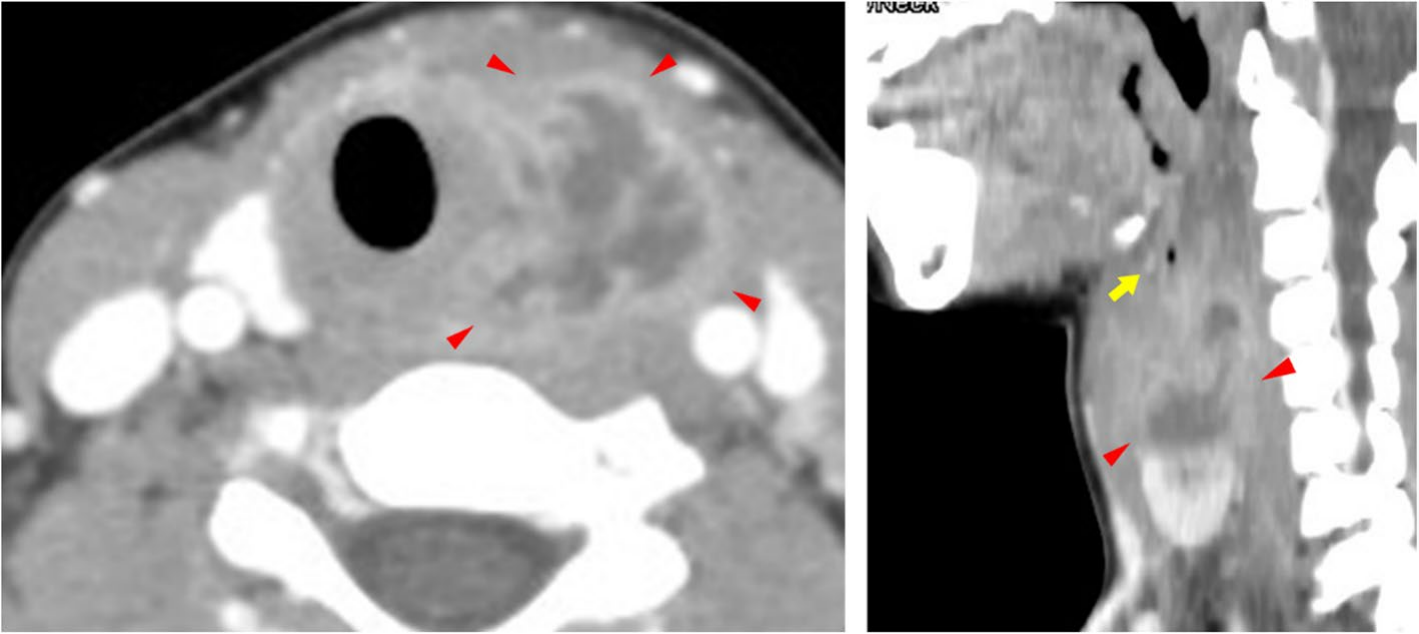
Contrast-enhanced computed tomography (CT) of the cervical region on admission. The contrast-enhanced CT scan of the cervical region revealed an abscess partially infiltrating the thyroid gland (arrowhead) and an air pocket (arrow)
